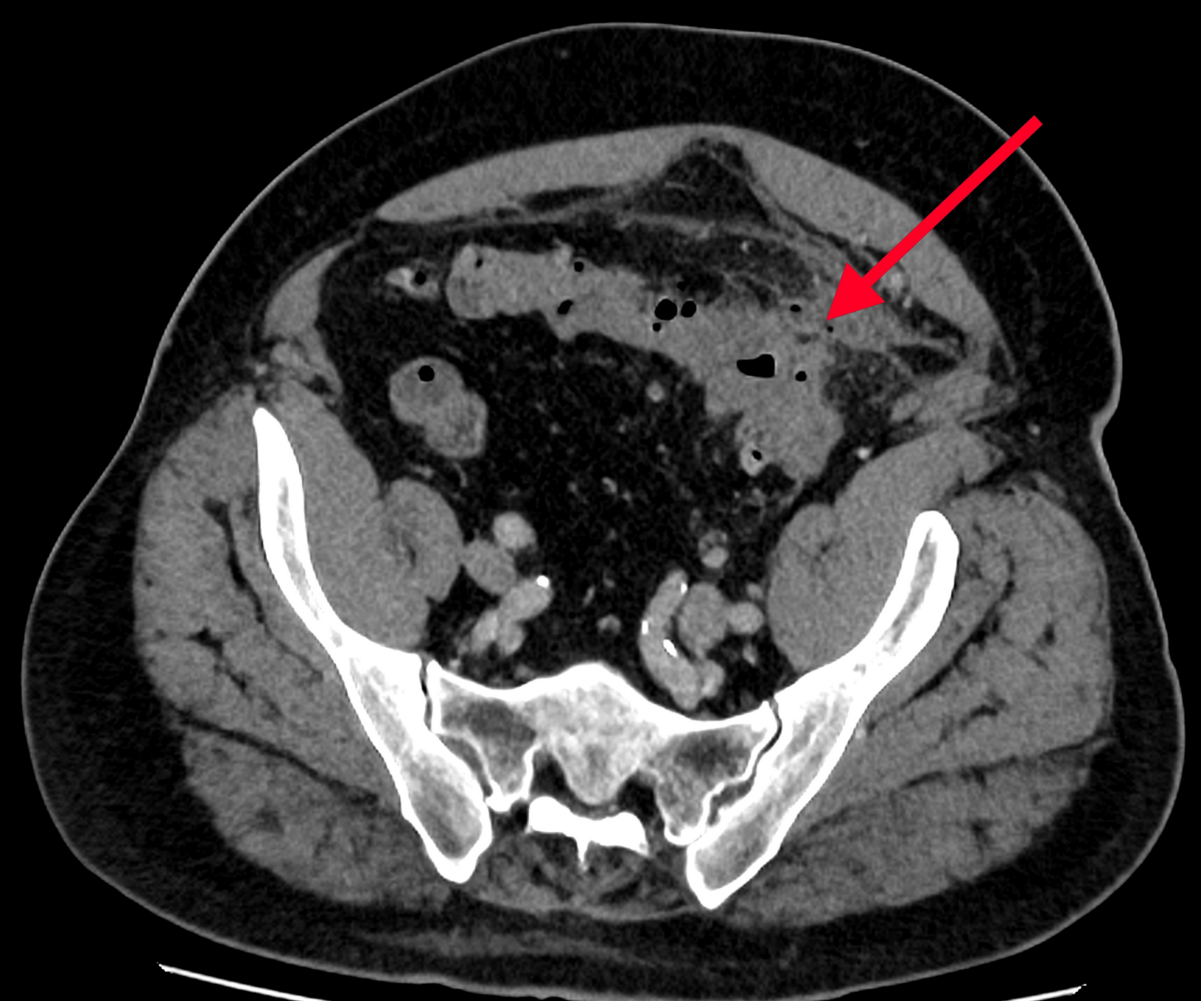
Axial view (inferior slice) of contrast-enhanced CT of the pelvis The red arrow highlights sigmoid diverticulitis with localized perforation and pericolic inflammation, representing the primary focus of disease responsible for the secondary small bowel changes.
